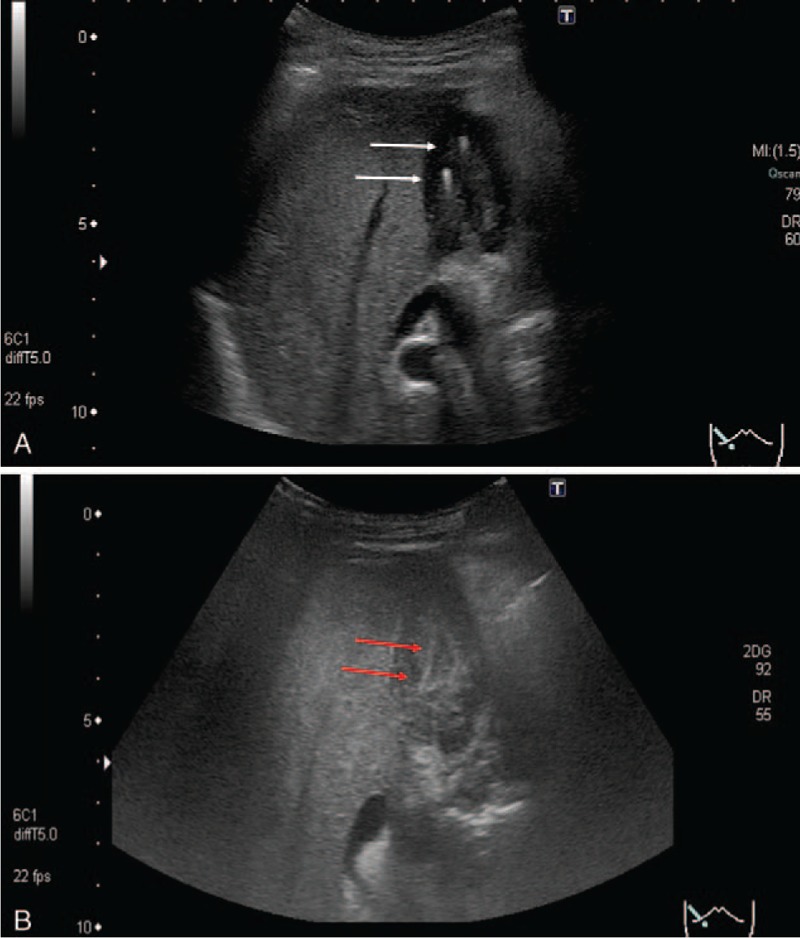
(A) Sonogram showed symmetrical wall thickening with echogenic foci (white arrow). (B) After intravenous Perflubutane injection, the inner layer of the gallbladder was enhanced (red arrow).
